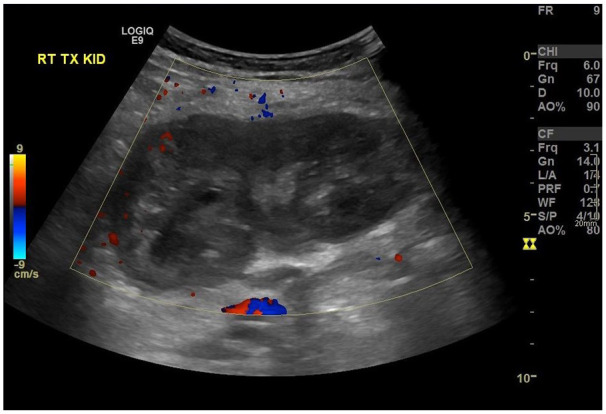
Initial ultrasound: Right iliac fossa transplant kidney, devoid of blood flow and oedematous.
